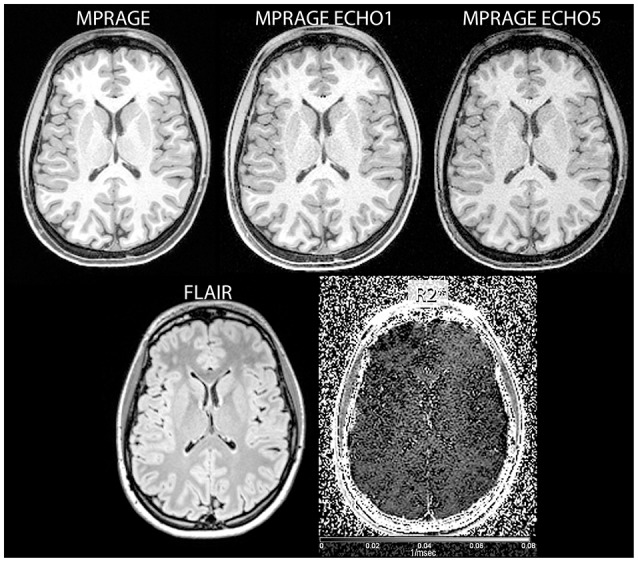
Examples of input images used in the study (clockwise from top left: reconstructed MPRAGE image, MPRAGE first echo, MPRAGE last echo, FLAIR, and ${\text{R}}_{2}^{*}$ images).
